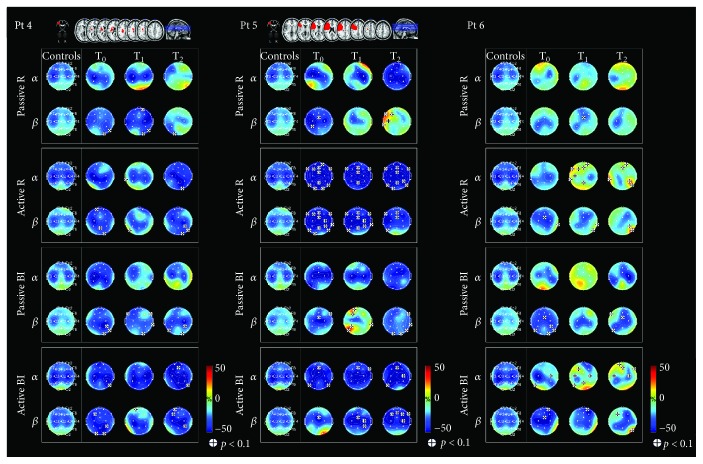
Lesions displayed on a magnetic resonance imaging brain template and topographic maps showing ERD/ERS values. ERD/ERS maps in the alpha and beta bands during passive and active movements with the affected hand and during bimanual passive and active movements (patient numbers 4, 5, and 6). Blue indicates maximal ERD. The *t*-test was applied individually for each patient in order to compare the ERD/ERS map of each patient to the mean ERD/ERS map of the controls (*p* < 0.1 (|*t*| > 1.895) indicated by (+)).
